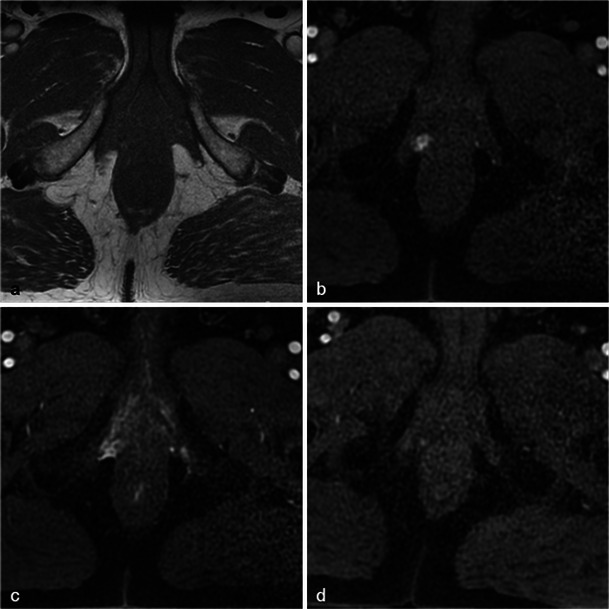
Suspected residual tumour. Day-7 post-treatment dynamic contrast-enhanced fat-saturated axial T1-weighted MR image showing early nodular enhancement in the prostate apex suggestive of residual tumour away from the treated area. Diffusion-weighted MRI was not contributive. The nodule was no longer visible on the 3-month post-treatment and 6-month post-treatment MRIs, and the 6-month post-treatment biopsy was negative
